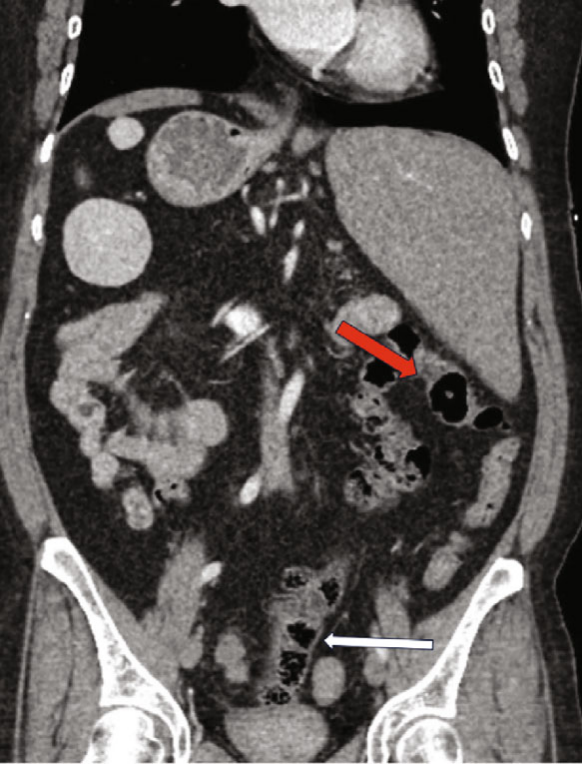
Coronal noncontrast CT demonstrates the midline position of the cecum and ascending colon (white arrow) and a short transverse colon present in the RUQ (red arrow).
